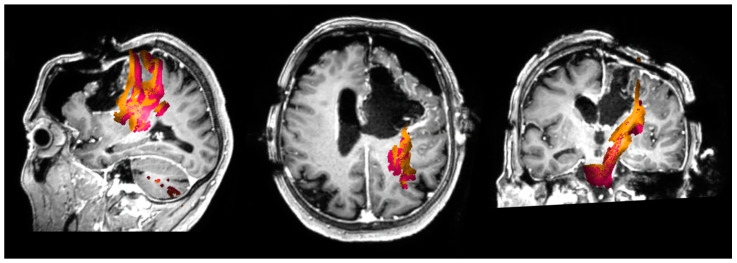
Navigated transcranial magnetic stimulation-based (nTMS) motor tractography corrected by intraoperative magnetic resonance imaging-based elastic fusion (IBEF)in an intraoperative magnetic resonance imaging (ioMRI)scan. This figure shows motor fiber tracts based on navigated transcranial magnetic stimulation (nTMS) in an intraoperative magnetic resonance imaging (ioMRI) scan in an exemplary patient case. The preoperative motor fiber tracts are shown in orange. The fiber tracts corrected by ioMRI-based elastic fusion (IBEF) are shown in pink.
